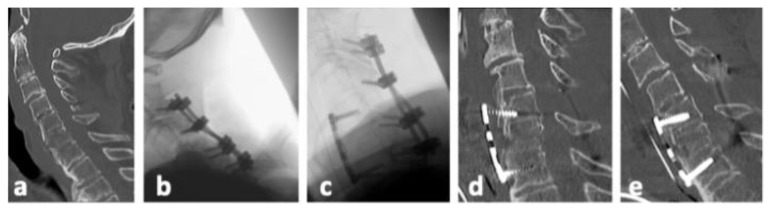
Representative CT images of patient no. 3 (Table 6). (a). Postinjury midline sagittal CT reconstruction. (b). Posterior stabilization from C3/4 to C6/7 with laminectomy of C5 due to spinal stenosis was performed. (c). Four days later anterior plate fixation C5 to C7 was added; no screws were placed in the fractured vertebral body of C6. (d). Secondary dislocation was detected in a CT scan 13 days after additional anterior fixation with loosening of posterior screws (not shown) and displacement of the anterior plate. (e). Combined revision was performed with posterior stabilization of C3/4/5/6/7 and anterior plate fixation C5 to C7.
